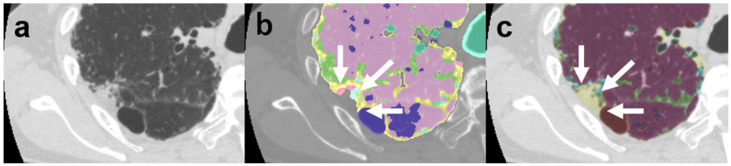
Images of a 72-year-old man with idiopathic pulmonary fibrosis. (a) Computed tomography image; (b) computer-aided diagnosis (CAD) method image; (c) deep-learning (DL)-based image. On the CAD method image (b), the pleuroparenchymal fibroelastosis (PPFE)-like lesion in the peripheral area (white arrow) was neglected because it was considered extrapulmonary. On the DL-based analysis image (c), the PPFE-like lesion (white arrow) is accurately classified as consolidation (CF_DL_). The color code follows that described in the legend of Figure 2.
